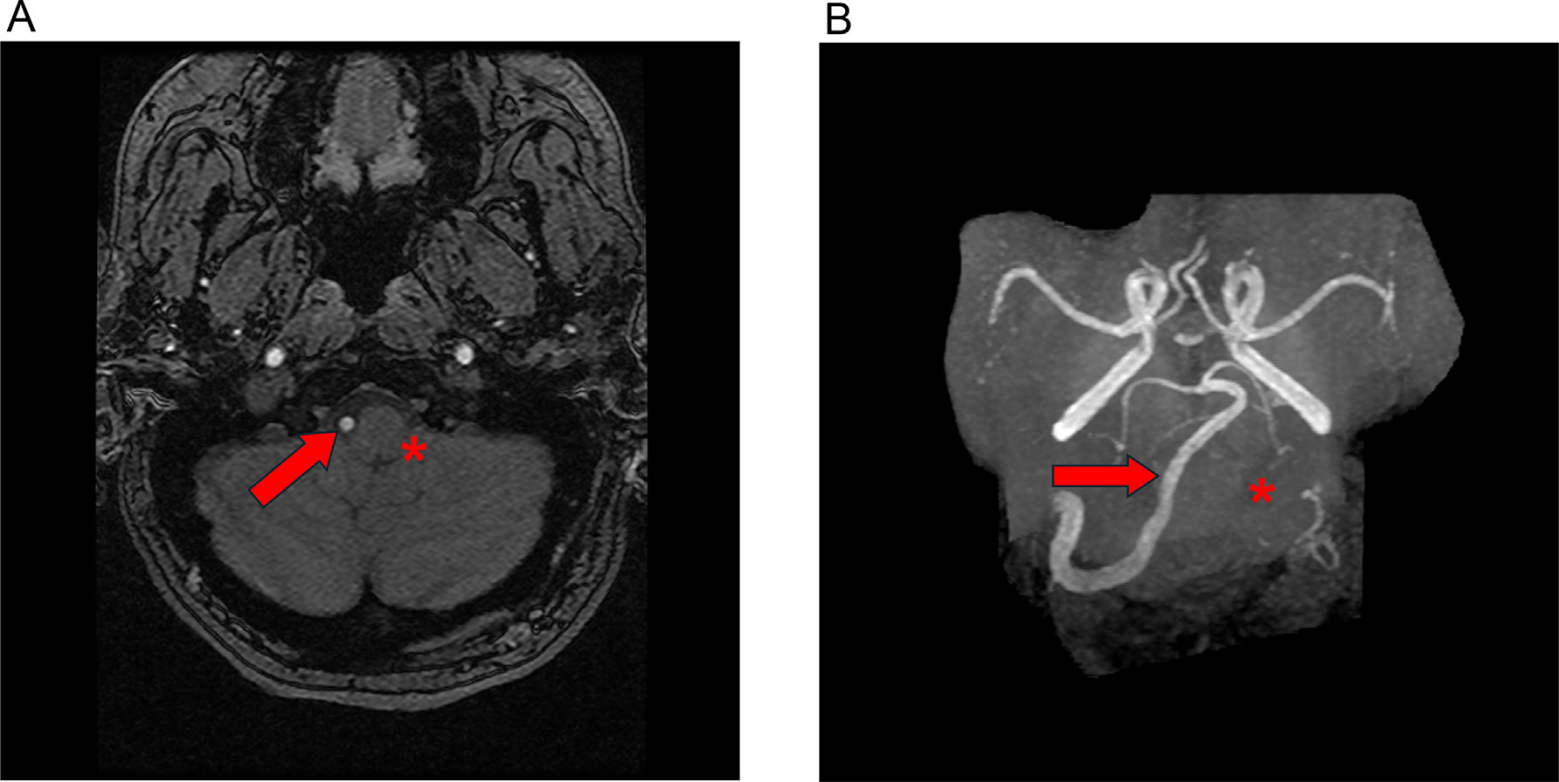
A, B. Head and neck MRI time of flight image and MRA coronal MIP reconstruction show an absent/hypoplastic left vertebral artery (red asterisk) with a normal contralateral right vertebral artery (red arrow).
